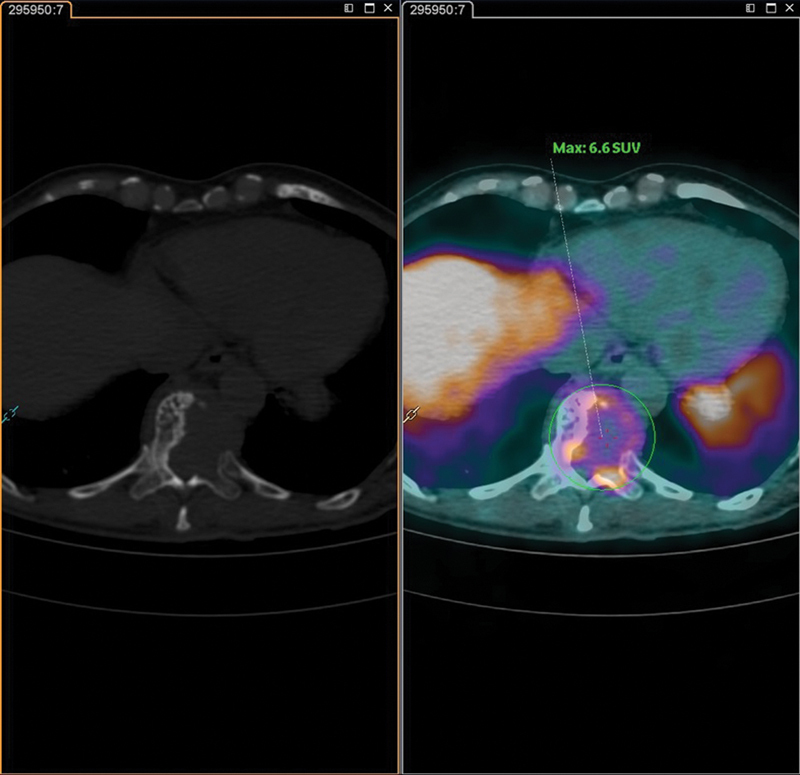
Axial computed tomography slices (
